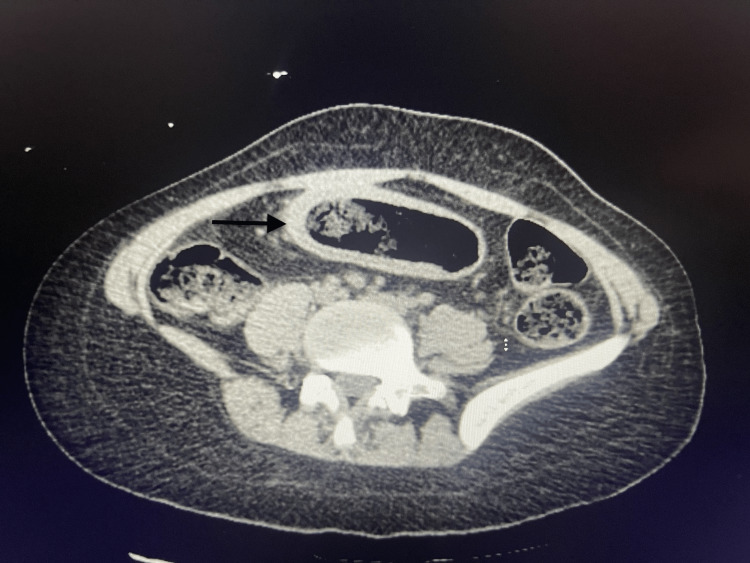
CT abdomen/pelvis without contrast showed stool throughout the colon with circumferential thickening (arrow) of the wall of the rectum and sigmoid colon likely proctocolitis. CT: computed tomography
